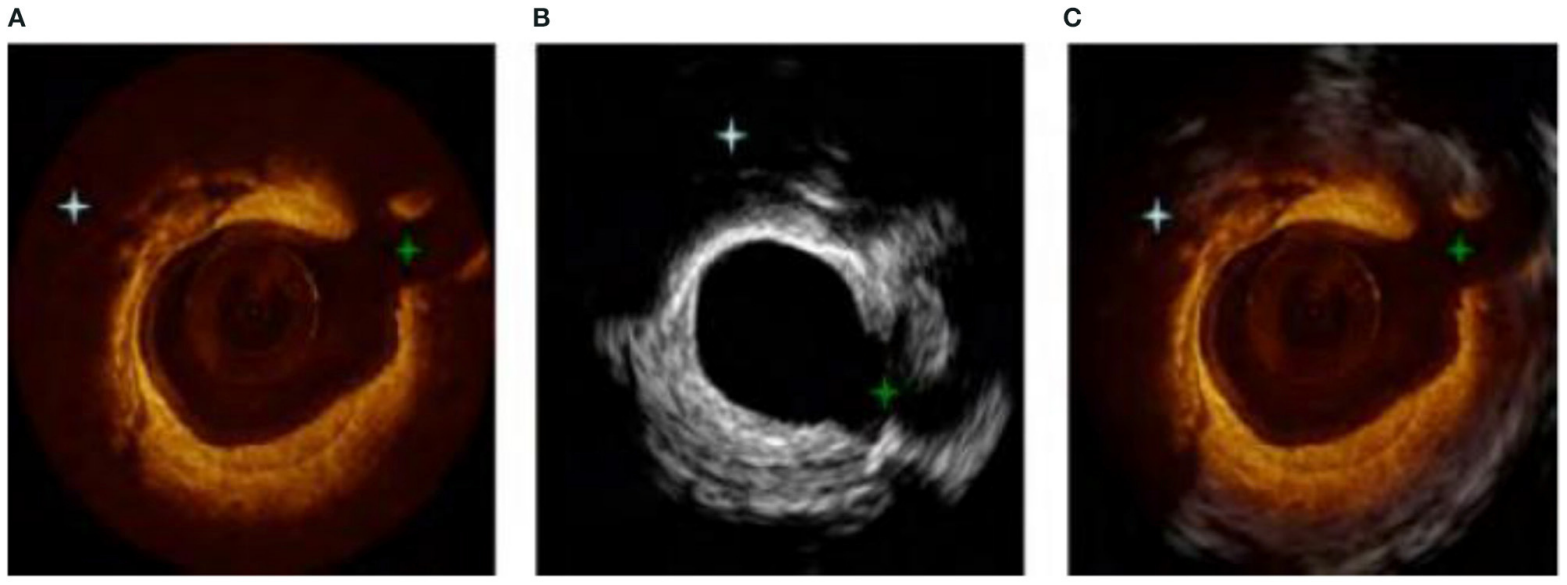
(A–C) Combination image of new intravascular ultrasound technology and IV-OCT.
